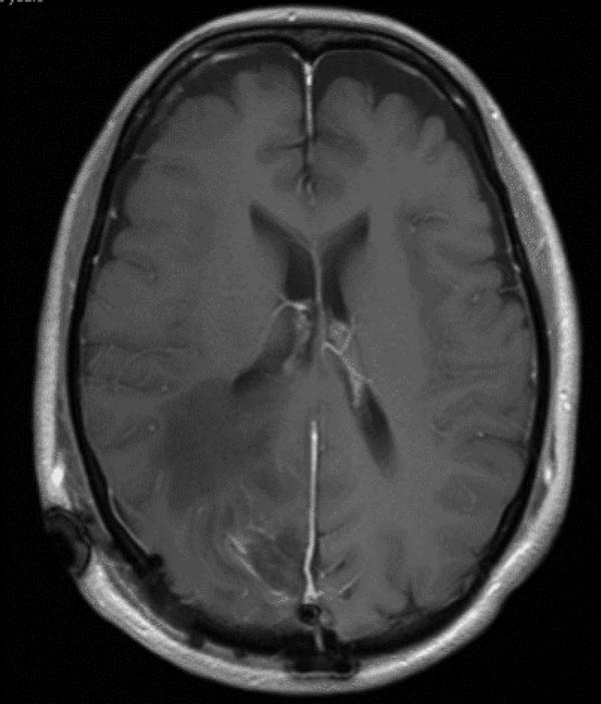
T1 weighted axial MRI postgadolinium enhancement within 48 h post-operatively. Small amount of enhancement within the surgical bed remains. Good resection margins with vasogenic oedema. This may represent a small residual. The rim-enhancing cystic lesions appear to have resolved.
